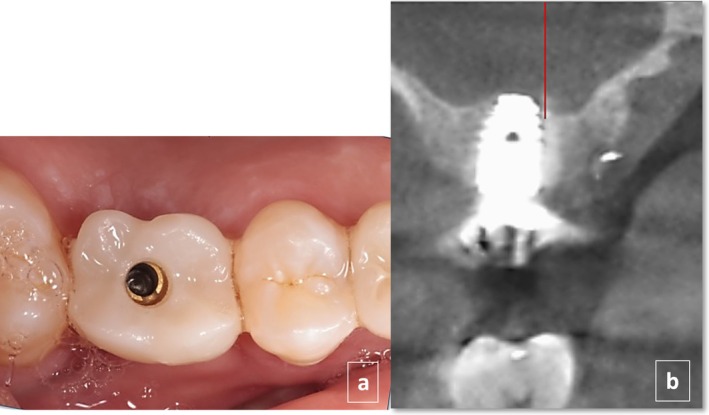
(a) Clinical view of the final zirconia crown; (b) CBCT coronal view showing bone growth with a follow‐up period of 2 years.
